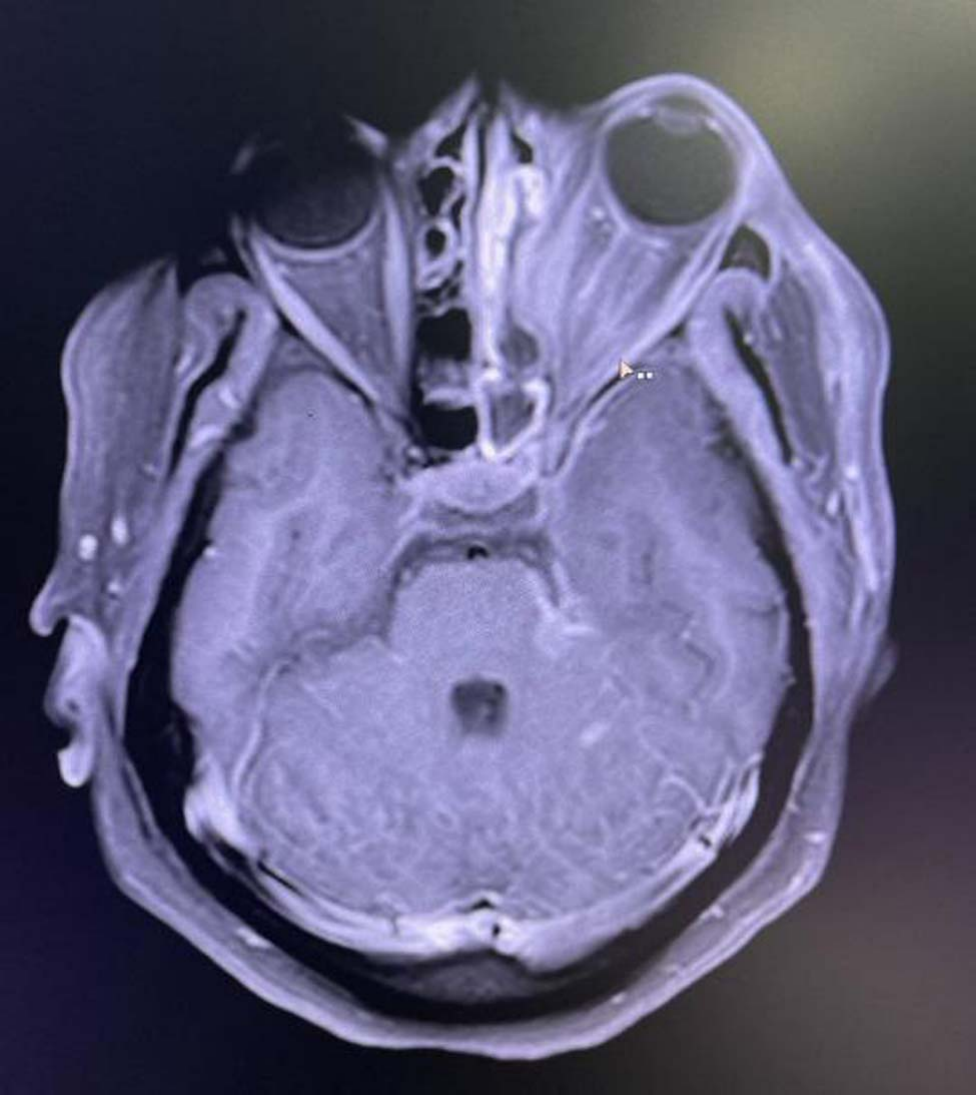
Post-contrast axial MRI showing heterogeneously enhancing soft tissue in the left ethmoidal and sphenoidal sinus with extension in the left nasal cavity. Also, enhancement was seen in extraocular muscles with mild anterior displacement of the left globe as well as in the left cerebellopontine angle along the left trigeminal nerve.
